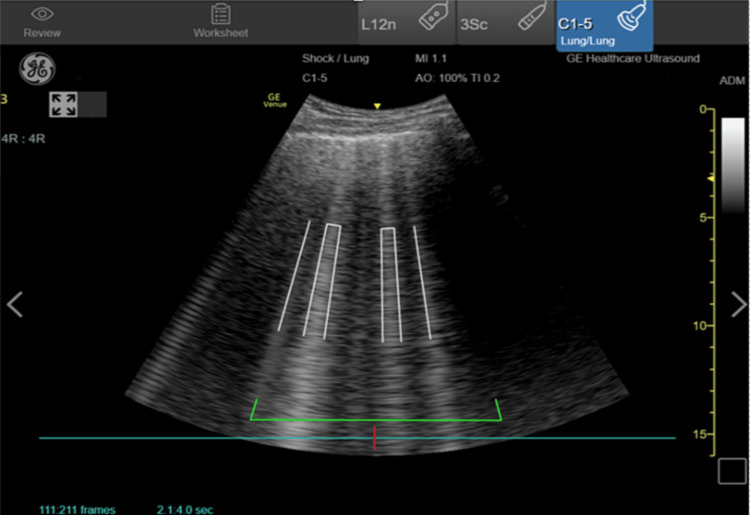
Point of care ultrasound with semi-automated software platform with B-lines
